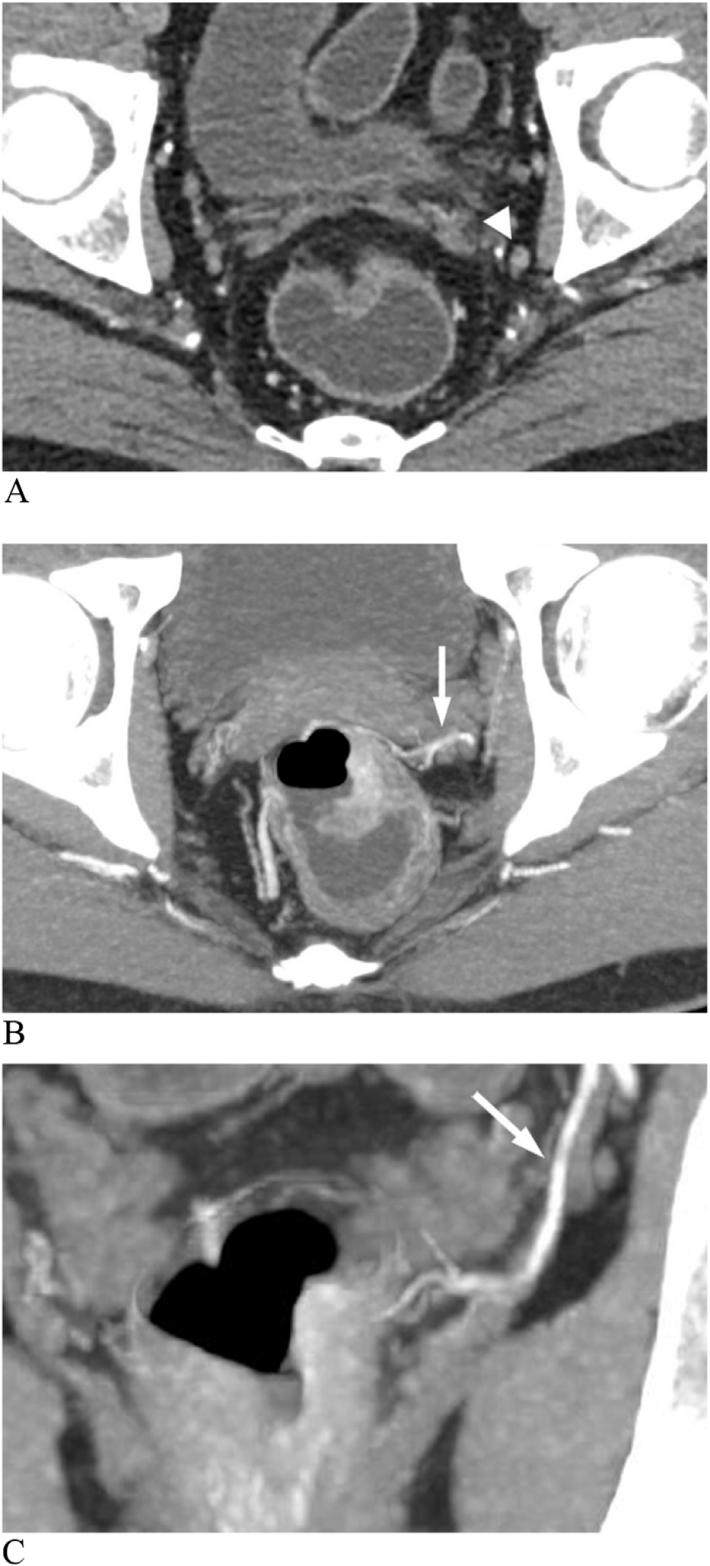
Enhanced CT images show a metastatic lateral lymph node (LLN) in rectal cancer. (A) Lymph node (arrowhead) with a short diameter of 5 mm. (B) Axial image shows an anterolateral middle rectal artery (MRA) (arrow). (C) Coronal image shows the left MRA (arrow) connecting the internal iliac artery to the thickened rectal wall.
